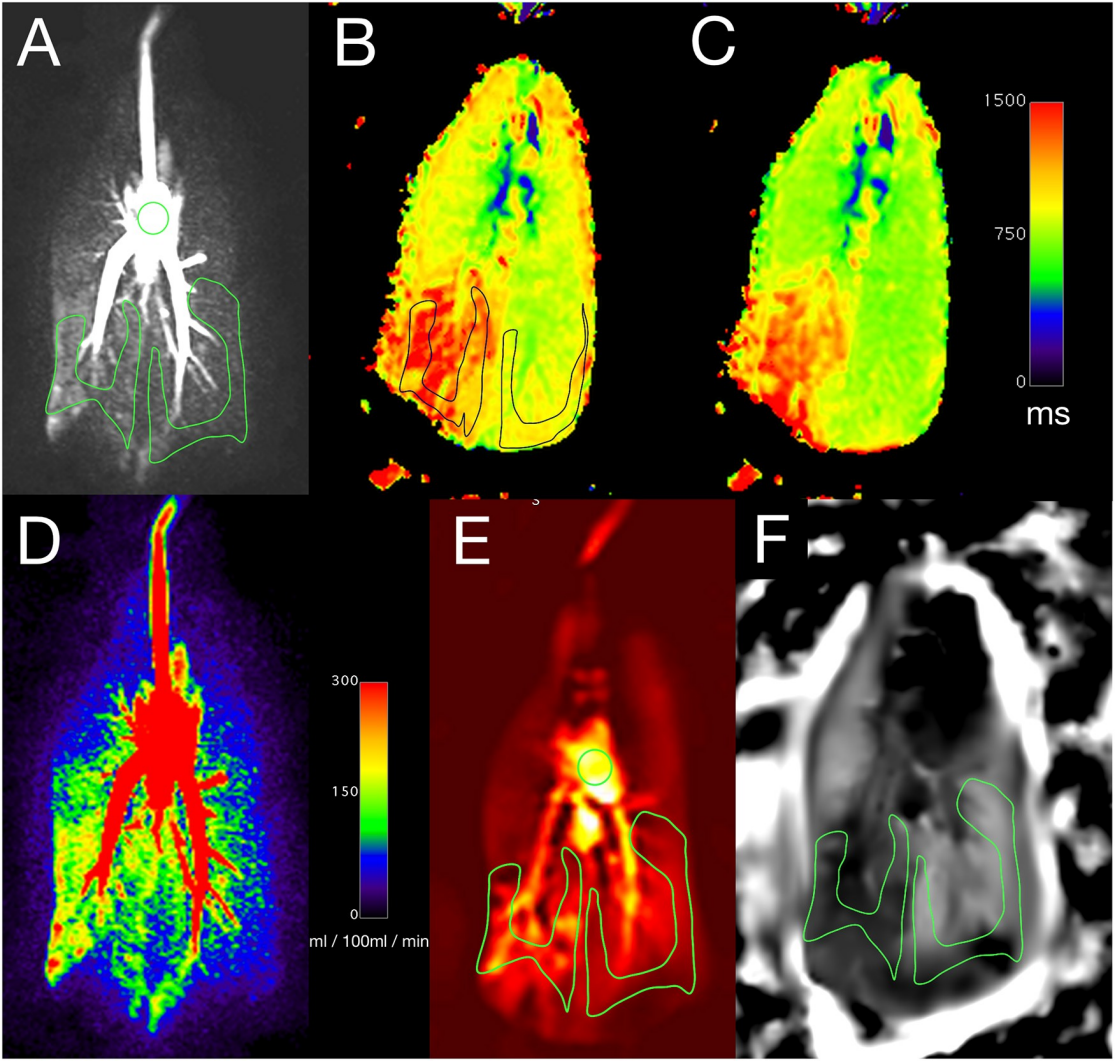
Functional MRI assessment. A Image of the dynamic contrast enhanced TWIST sequence with ROIs in the distal parenchyma of right and left lower lobe. The additional ROI in the central pulmonary artery was used for normalization of the perfusion weighted signal of Fourier Decomposition. Corresponding slices for oxygen enhanced T1 maps under B F_iO2_ = 0.21 and C F_iO2_ = 1.0. D Parenchymal perfusion map derived from DCE. E Perfusion weighted Fourier Decomposition with ROI placement and F Ventilation weighted Fourier Decomposition.
